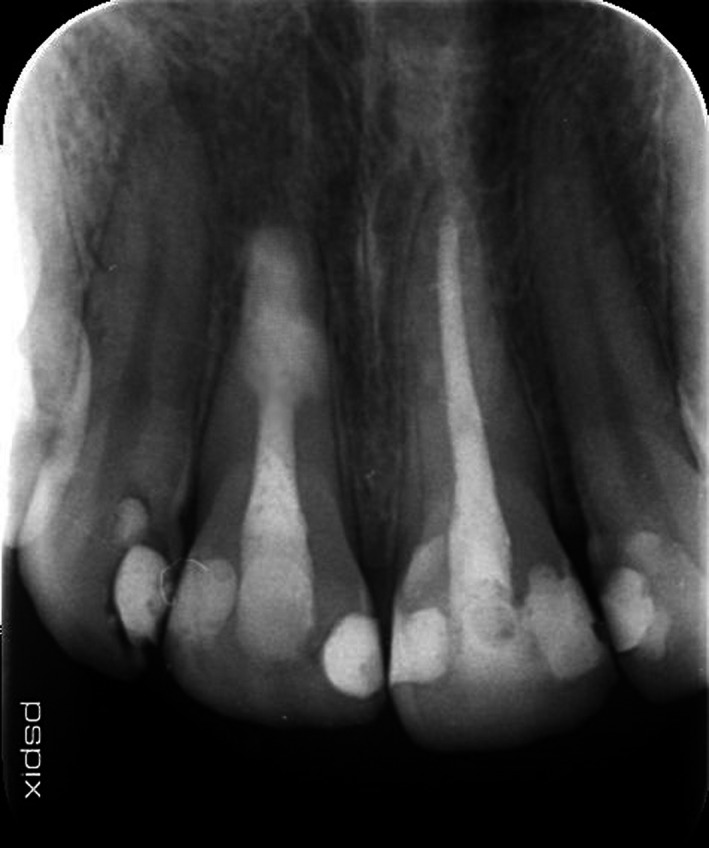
Follow‐up radiograph after 12 mo
